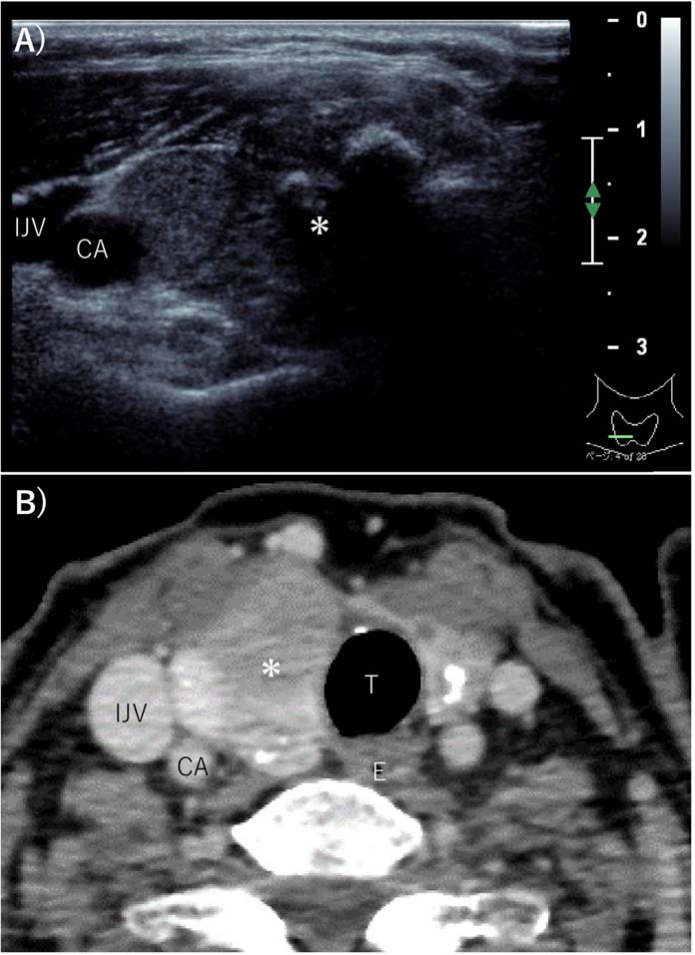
Pre-operative imaging findings of papillary thyroid cancer. (A) Ultrasonography revealed a hypoechoic 28-mm mass with internal calcification was found in the right lobe of the thyroid gland. (B) Contrast-enhanced CT revealed a 37 mm× 25 mm tumor in the right lobe of the thyroid gland without surrounding invasion and no lymph node metastasis or distant metastasis. ^*^: tumor, CA: carotid artery, IJV: internal jugular vein, T: trachea, E: esophagus.
